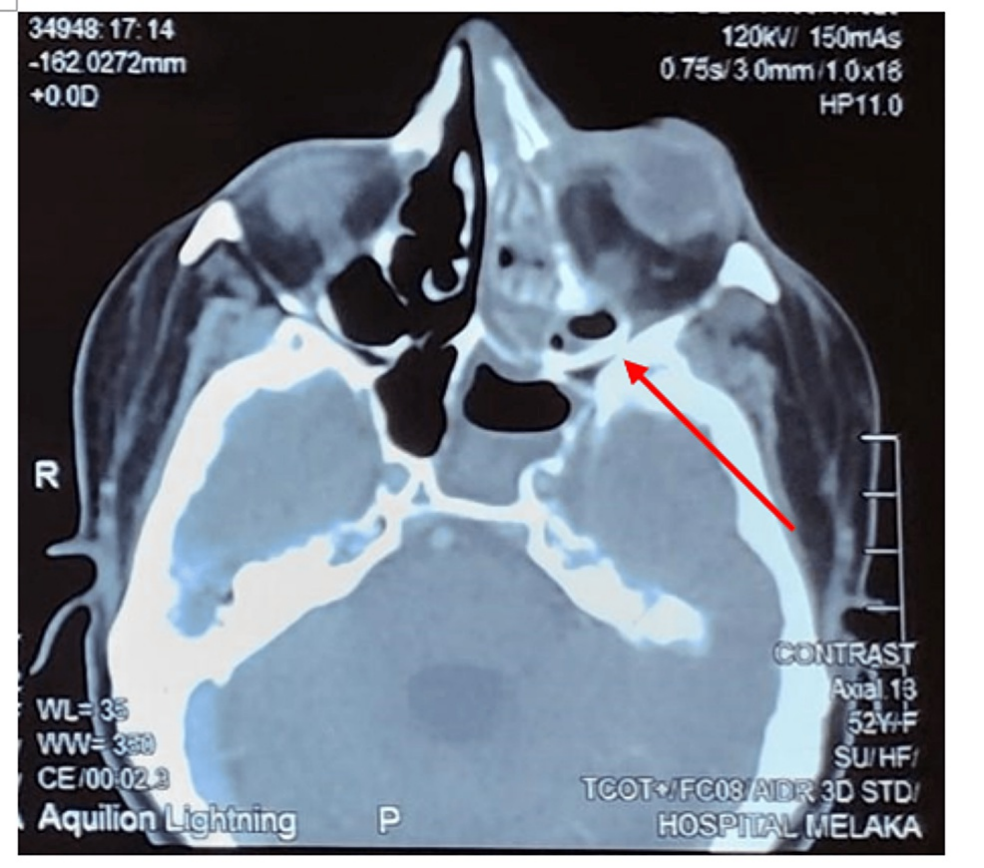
Contrast-enhanced computed tomography axial section showed mucosal thickening within the left frontal, ethmoid, maxillary, and sphenoid sinuses with involvement of the left orbit.
